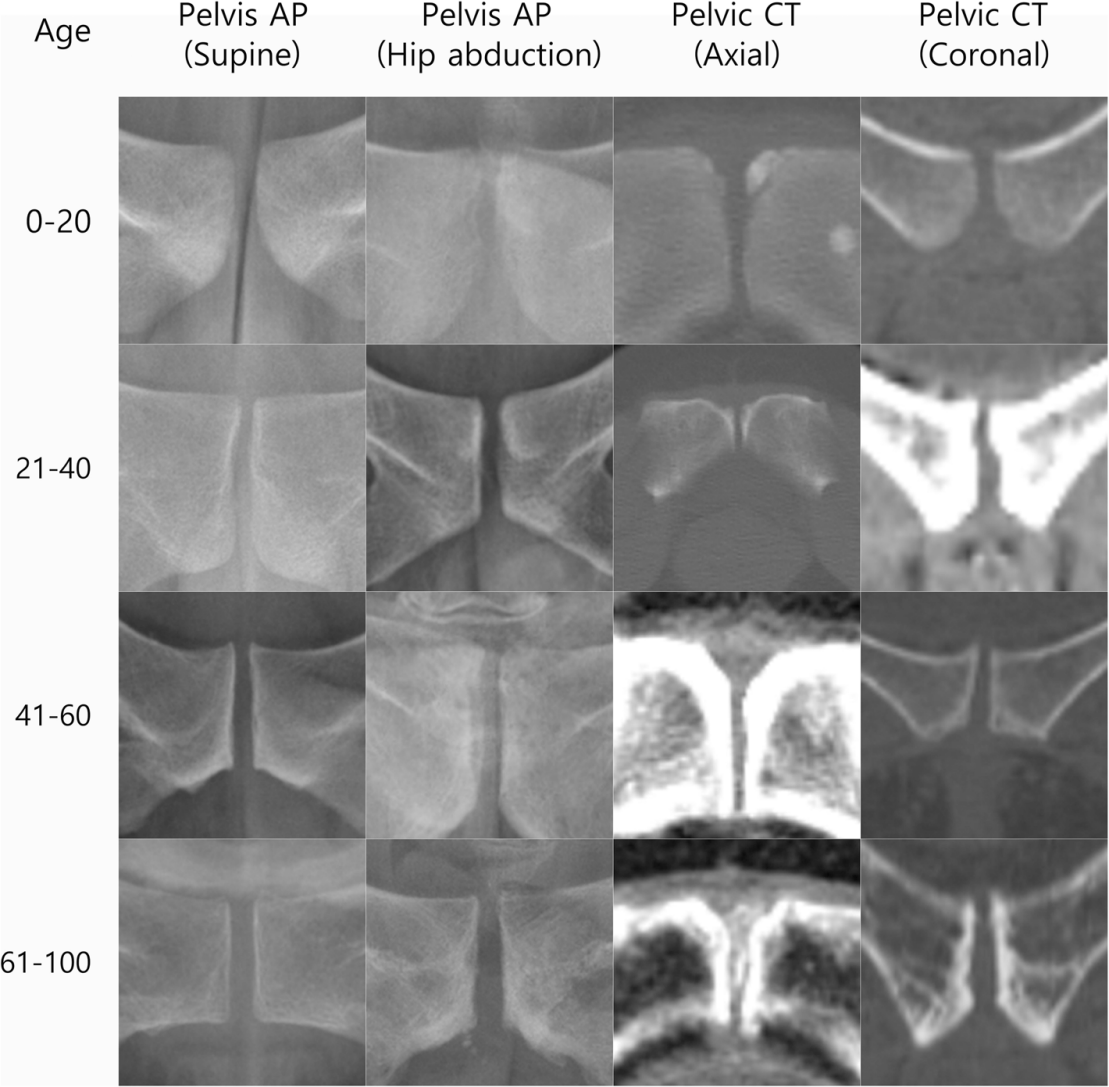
Width of pubic symphysis relating to age in each view. First column: pelvis AP (supine), second column: pelvis AP (hip abduction view), third column: pelvic CT (axial view), fourth column: pelvic CT (coronal view), first raw: 0–20 years, second raw: 21–40 years, third raw: 41–60 years, fourth raw: 61–100 years
